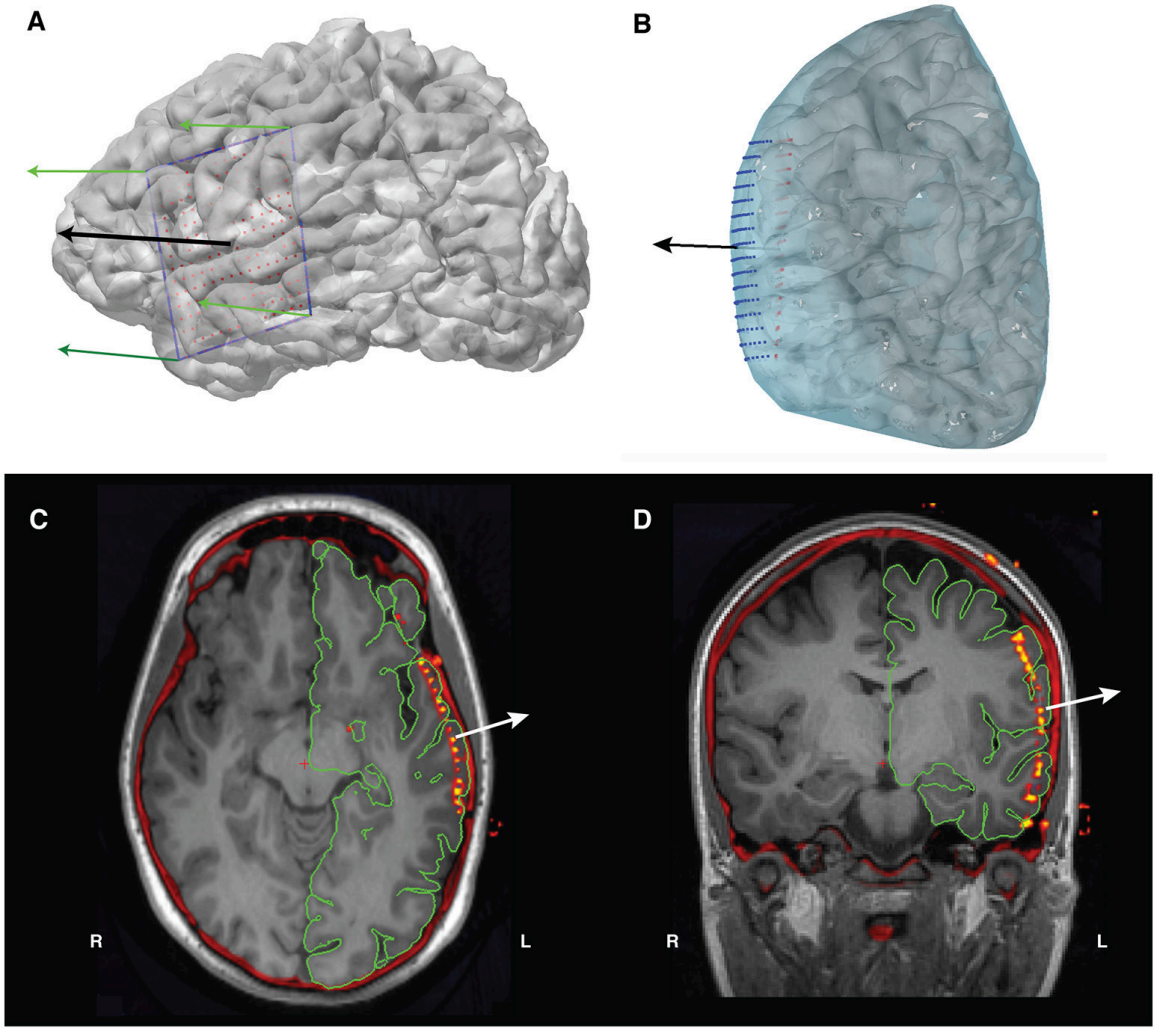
(A) The grid's corner electrodes are manually located. We interpolate the locations of the rest of the grid electrodes using these corner coordinates, giving us the electrode grid shown in red. The green arrows are the four normal vectors calculated from the corners, and the black arrow is the mean of those normal vectors and will act as our projection direction. (B) Projection of the interpolated grid (red) to the convex hull of the pial surface (blue) using the mean normal vector (black arrow). The final projected electrode grid is shown in blue. (C) Axial and (D) coronal views of the co-registered CT overlaid on the T1 MRI, showing the location of the electrodes prior to surface projection.
